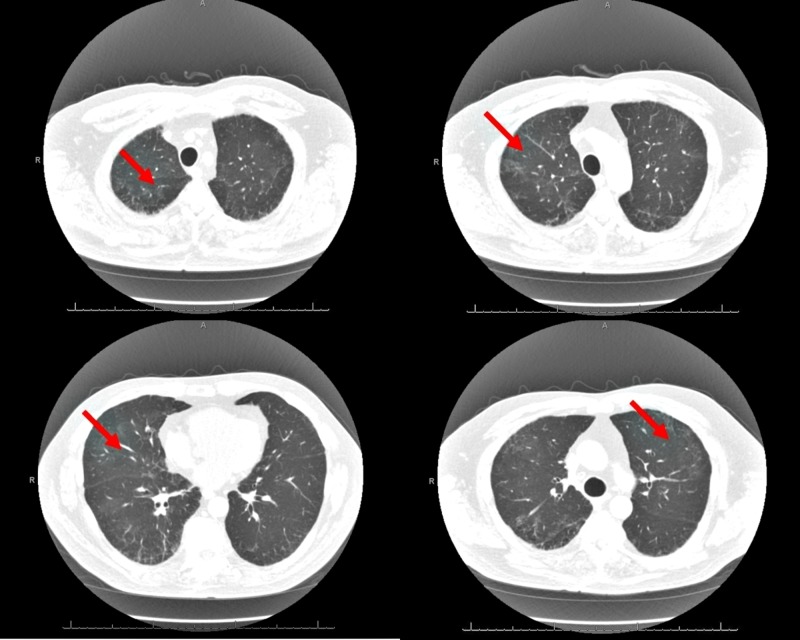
Computerized tomography (CT) scan of chest at follow-up visit three weeks post-treatment showing resolution of pulmonary infiltrates as indicated by red arrows
